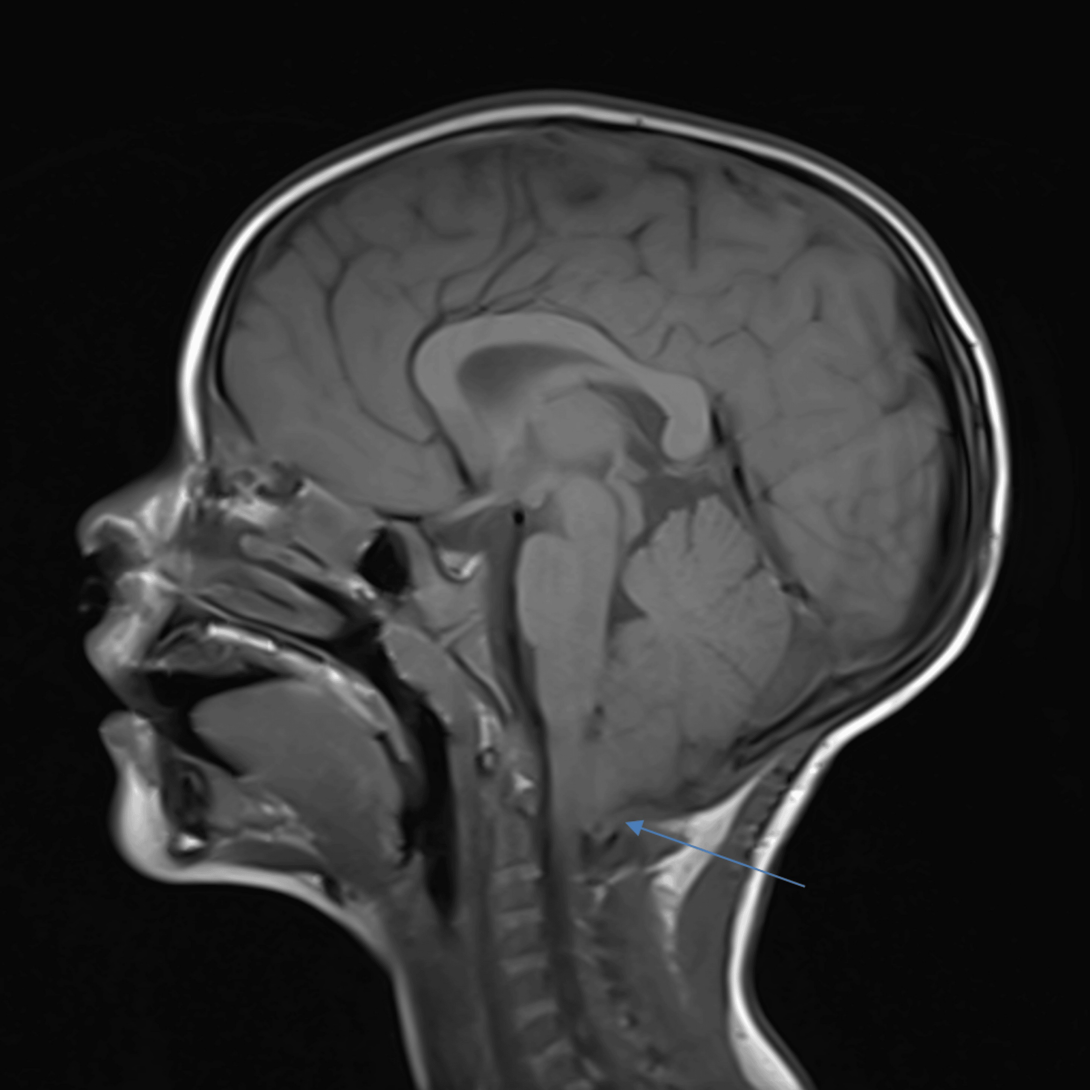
Sagittal T1 MRI imaging of the proband post surgery, demonstrating the expected postoperative changes of a suboccipital craniotomy for Chiari decompression. The blue arrow shows persistent narrowing of the cisterns at the craniocervical junction, unchanged from an earlier MRI two months prior.
